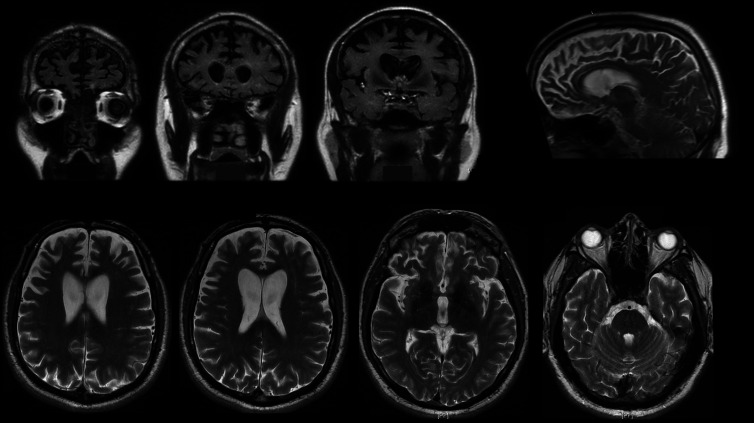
MRI. Coronal FLAIR (fluid-attenuated inversion recovery) sequences and sagittal T2-weighted imaging (top row) showed widespread lateral and medial frontal atrophy. Axial T2 images (bottom row) also showed symmetrical dilatation of the frontal horns of the lateral ventricles.
